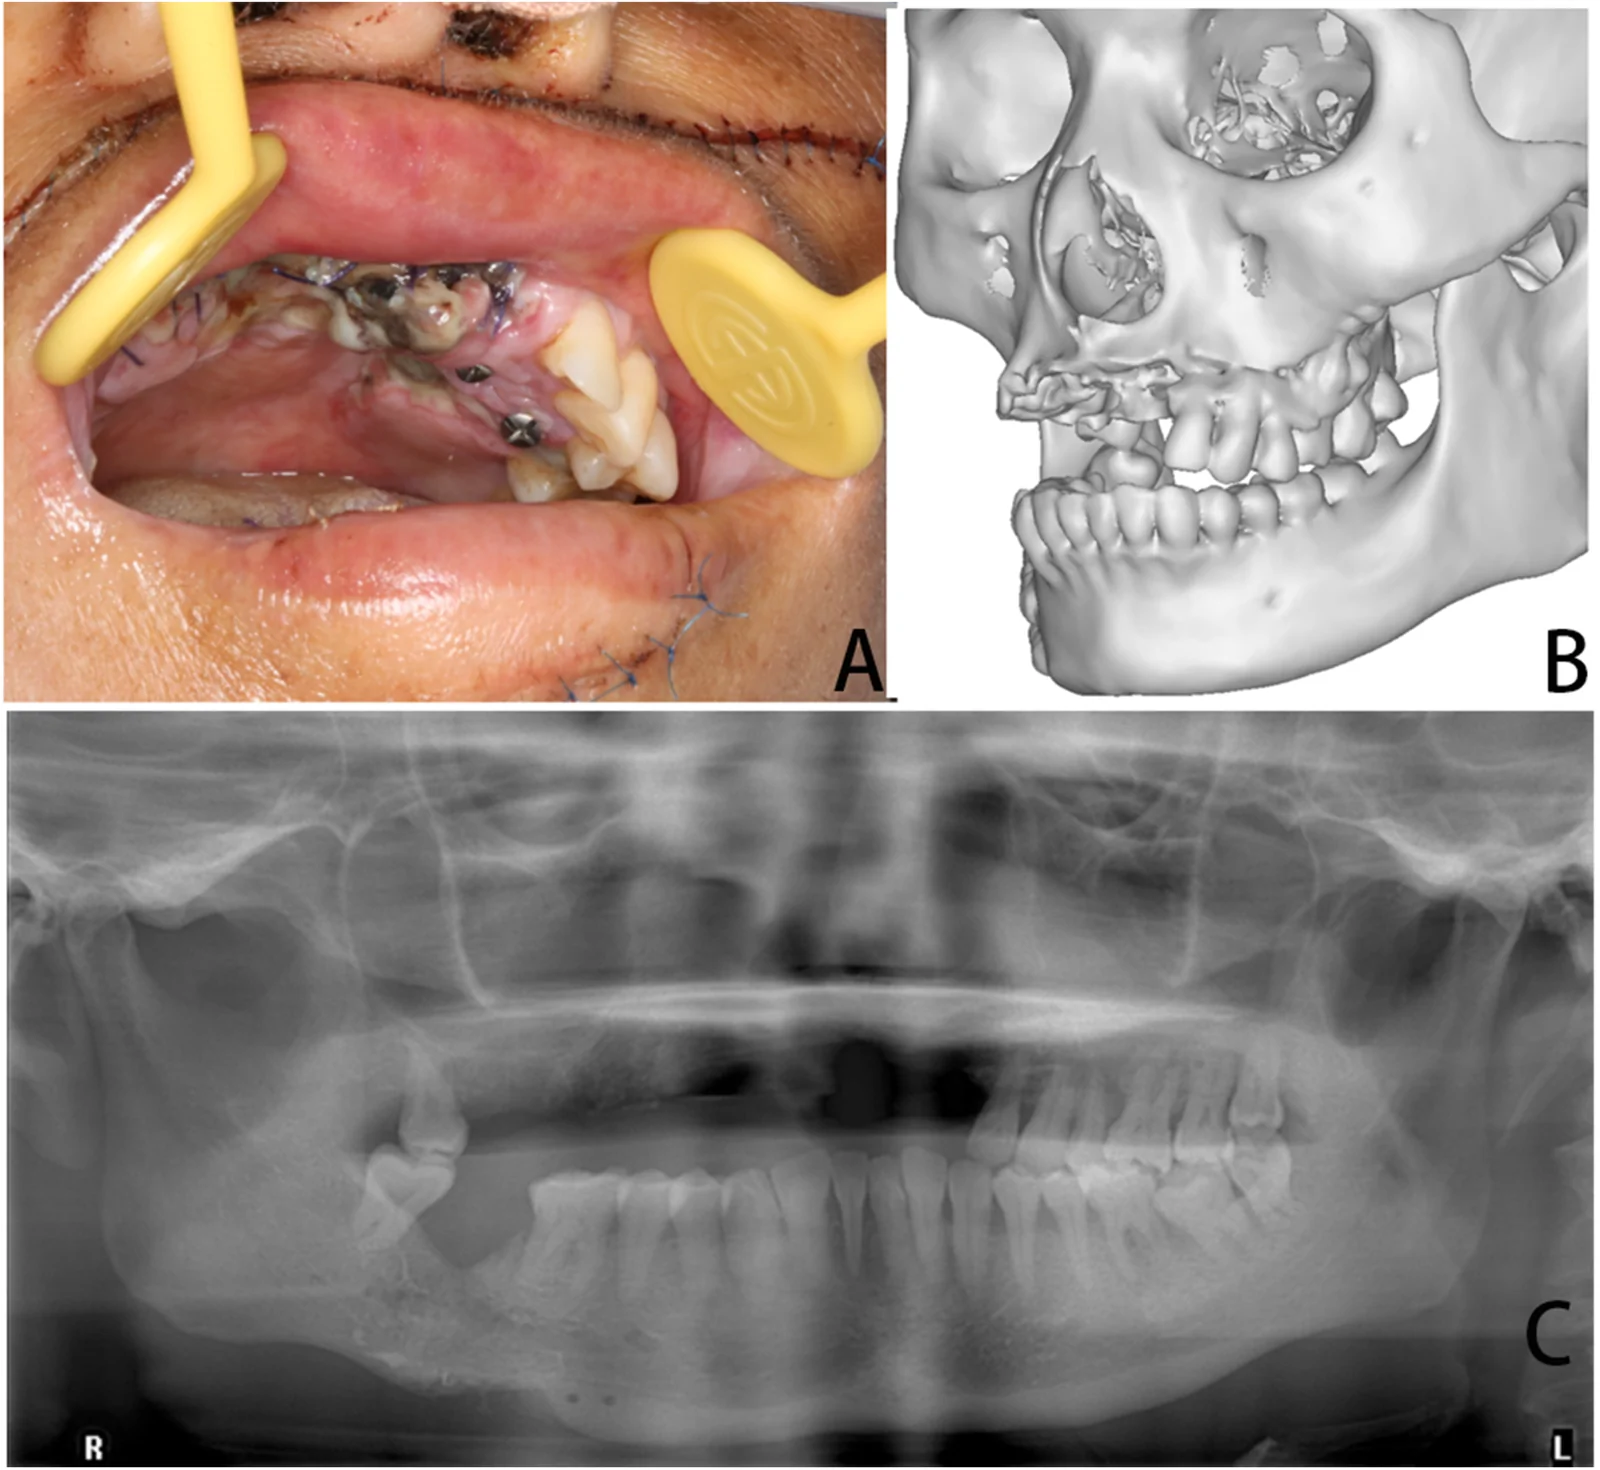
Postoperative and follow-up images of case 2. (A) Follow-up intraoral photograph. (B) Postoperative three-dimensional CT reconstruction. (C) Six-month panoramic radiograph.
